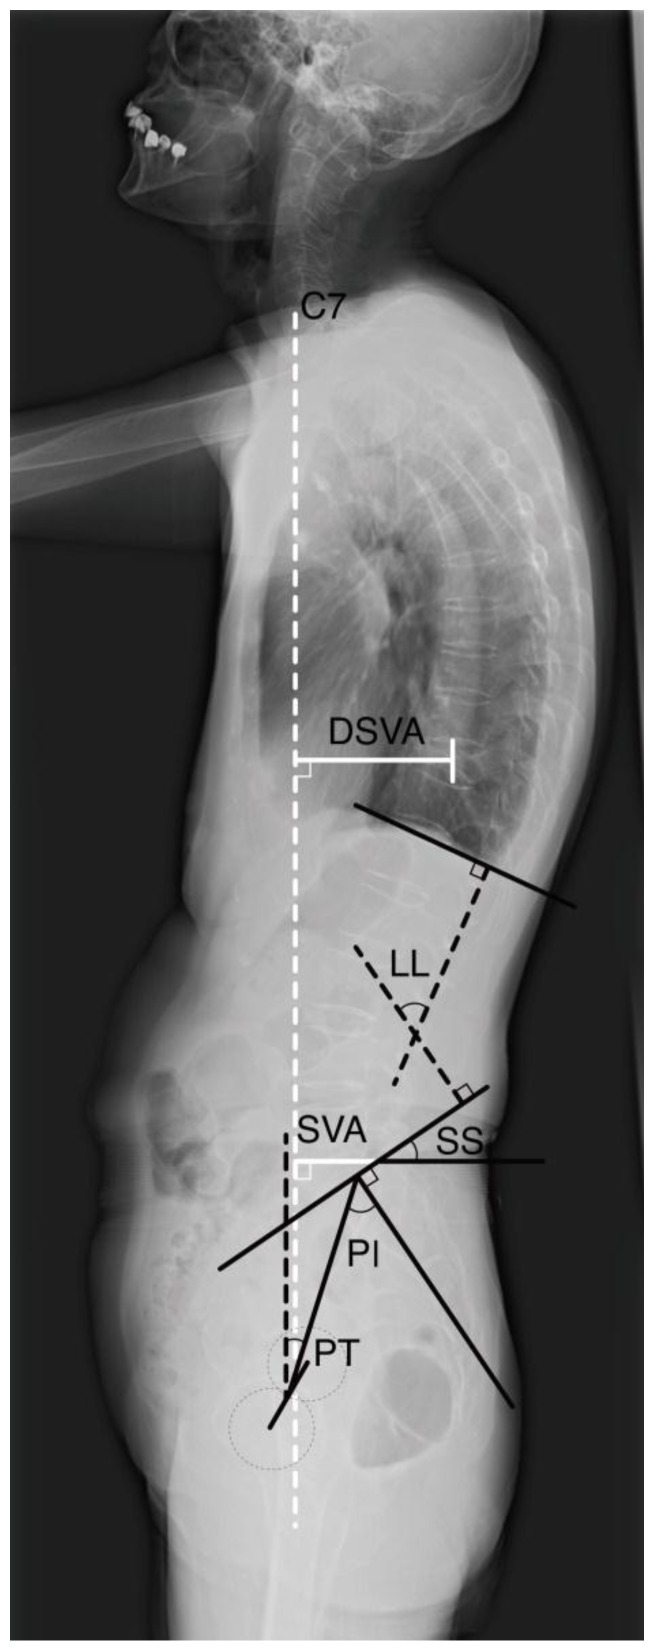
Measurement of spinopelvic parameters, sagittal vertical axis (SVA) and center of the fractured vertebra (DSVA). Owing to the nature and deviation of the spine lateral plain films, we defined the center of the femoral head used in the measurement of this study as the midpoint between femoral head of bilateral side. PI was defined as the angle between the vertical line of the sacral plateau and the line drawn from the mid-sacral plateau to the center of the femoral head. PT was defined as the angle between a line drawn from the mid-sacral plateau to the center of the femoral head and plumb line of the center of the femoral head. SS was defined as the angle between the sacral plateau and a horizontal line. Following Cobb’s method, LL was defined as the angle between the superior endplates of the L1 and S1 vertebrae. SVA was defined as the distance from the posterosuperior corner of S1 to the C7 plumb line. DSVA was defined as the distance between the C7 plumb line and the center of the fractured vertebra.
